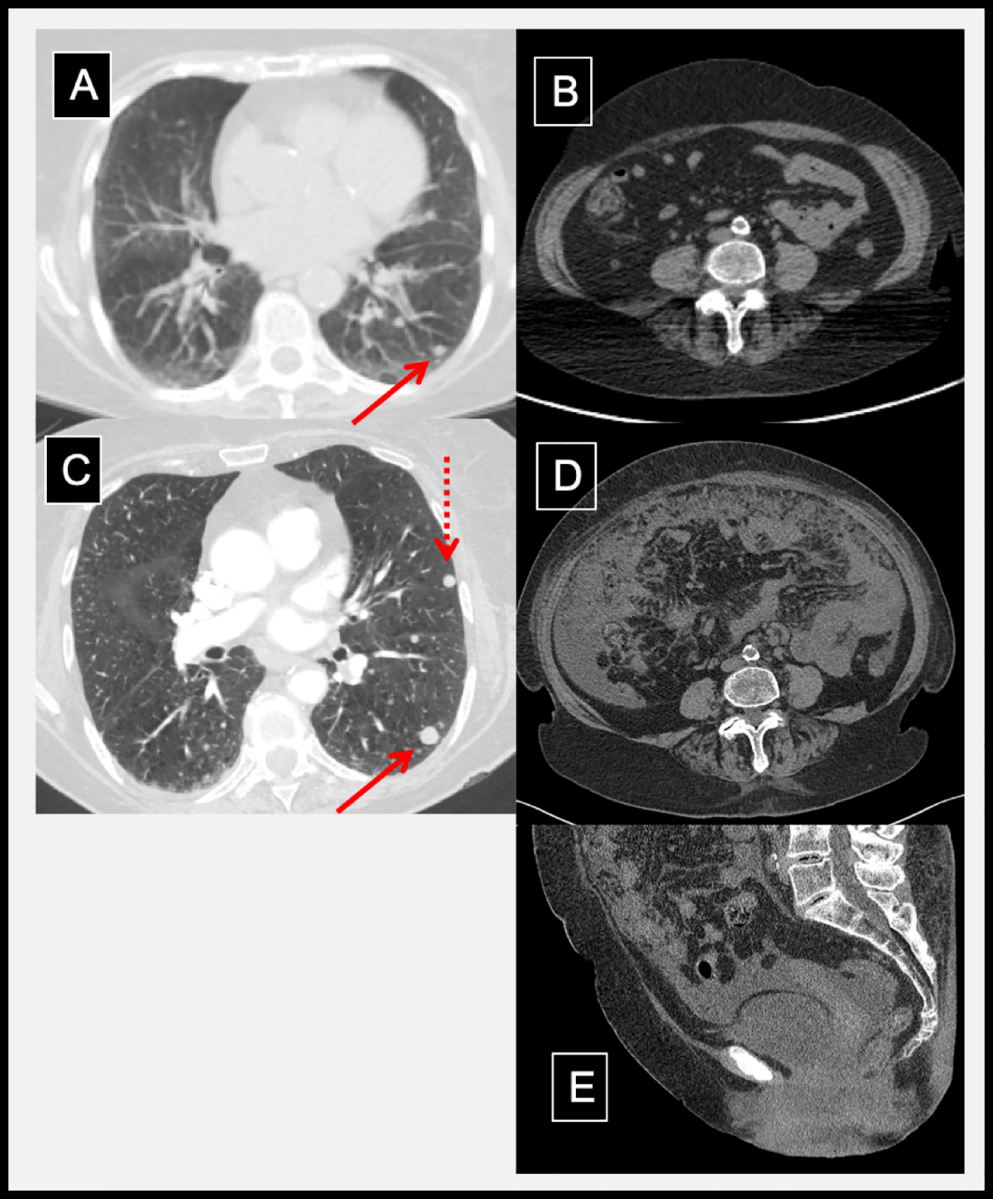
Radiographic evidence of hyperprogression and inflammation following dual immune checkpoint inhibition. Pre-treatment CT chest with a lung metastasis (A); solid arrow) and a normal abdominal CT (B). Three weeks after ipilimumab and nivolumab, prior lung metastasis grew (C; solid arrow) and new metastasis developed (C; dotted arrow). In addition, abdominal distention, omental caking, peritoneal metastases, and pelvic ascites developed as shown in axial (D) and mid-sagittal (E) abdominal/pelvic CT.
